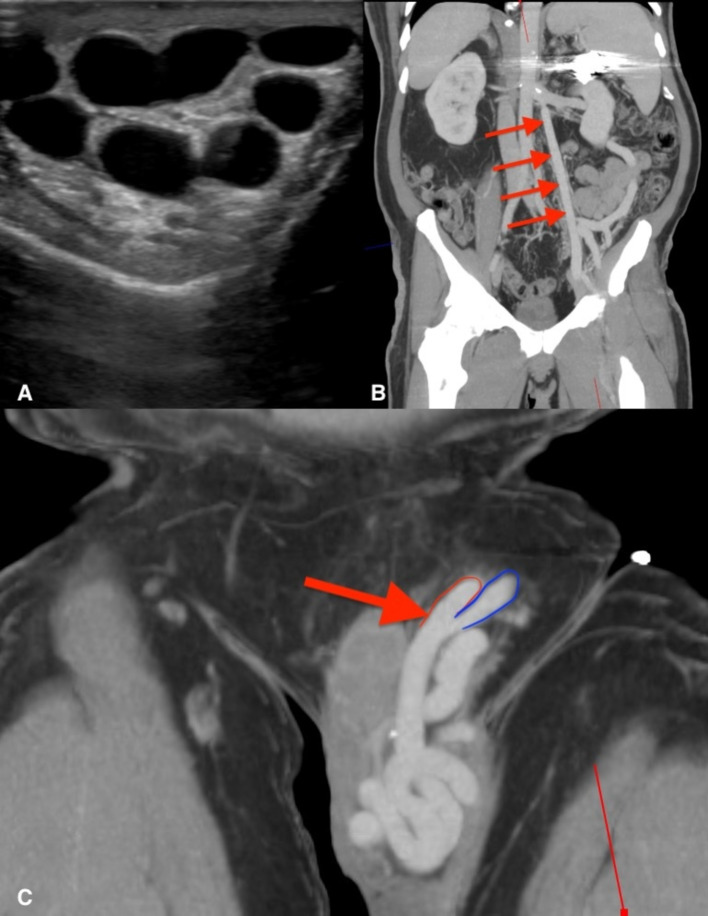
A A transabdominal ultrasound demonstrated complex scrotal venous anatomy. B On coronal view, a dilated left gonadal vein can be seen draining into the left renal vein (red arrows). C The confluence (red arrow) of the sigmoid and left colic vein branches (red and blue tracings) is seen within the scrotum with complex venous flow
